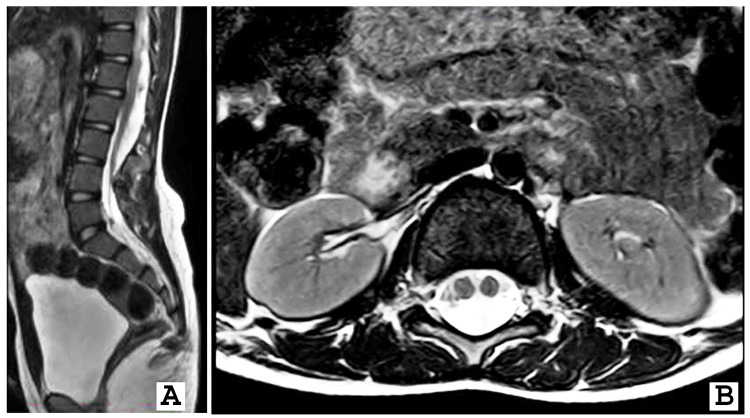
A and B, magnetic resonance imaging showing T2WI image with two spinal cords within the same dural sac-type 2 diastematomyelia at all L3-L5 levels T2WI: T2 weighted imaging
